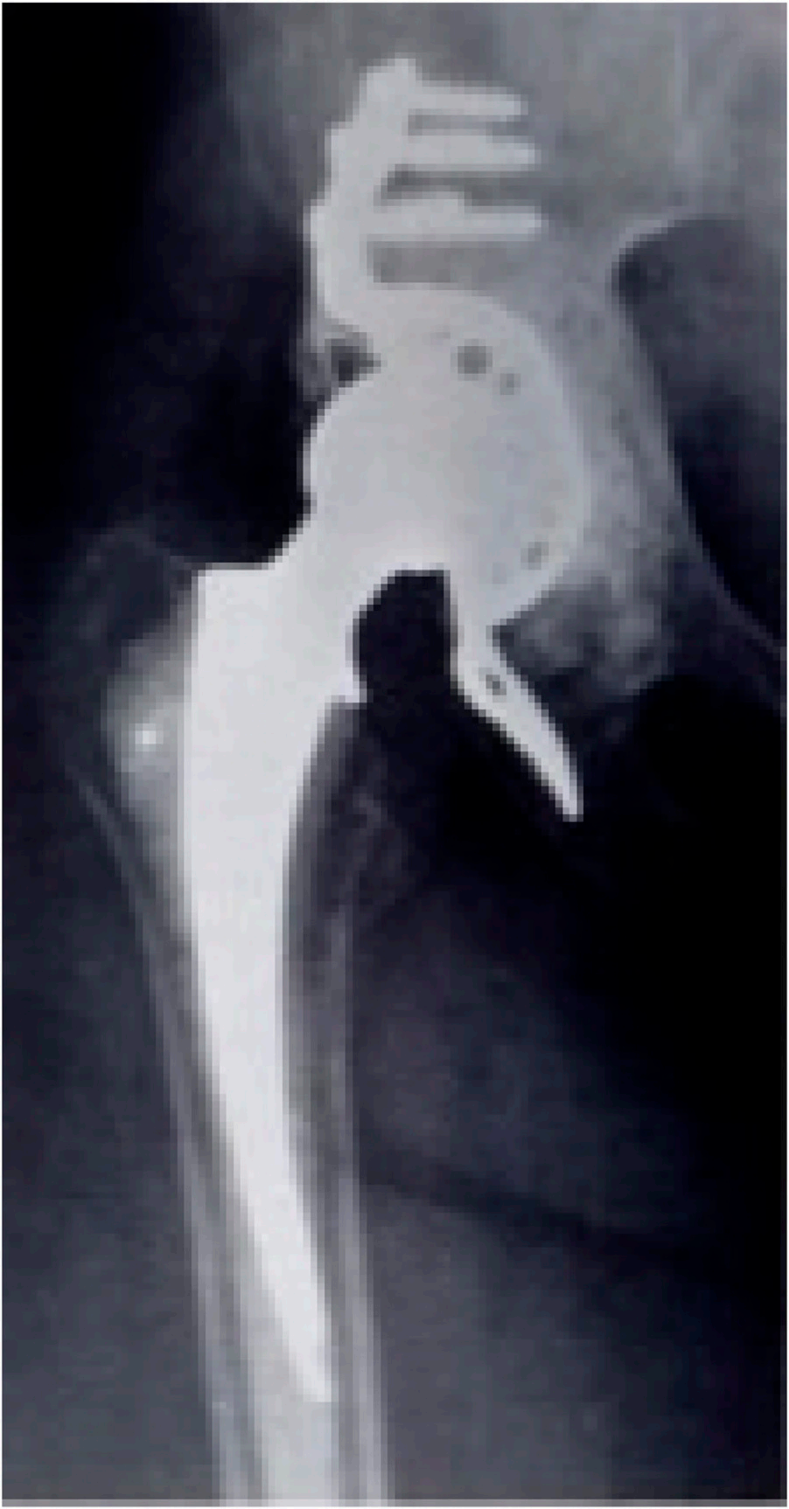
Radiograph of a patient’s right hip with a BS cage (Rosson and Schatzker, 1992).
